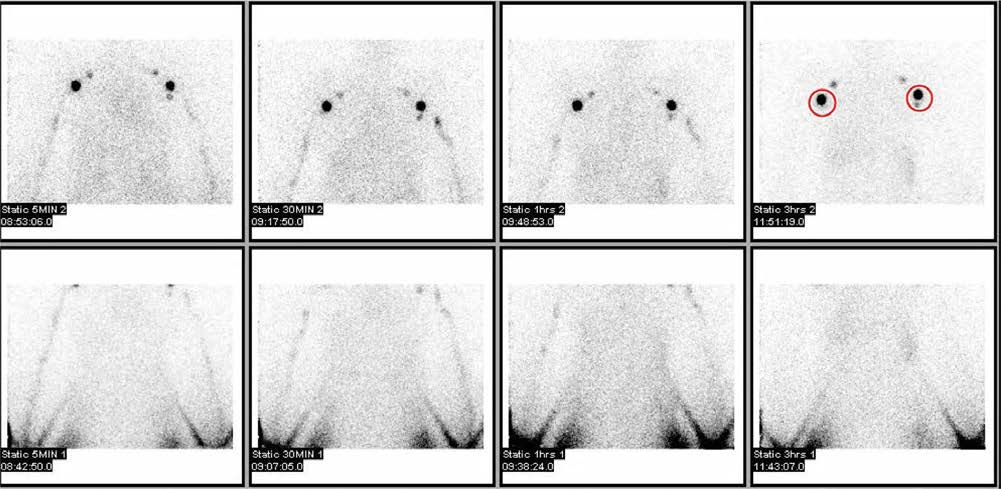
Normal lymphoscintigraphy. There are axillary lymph node uptakes on both side in circles.
